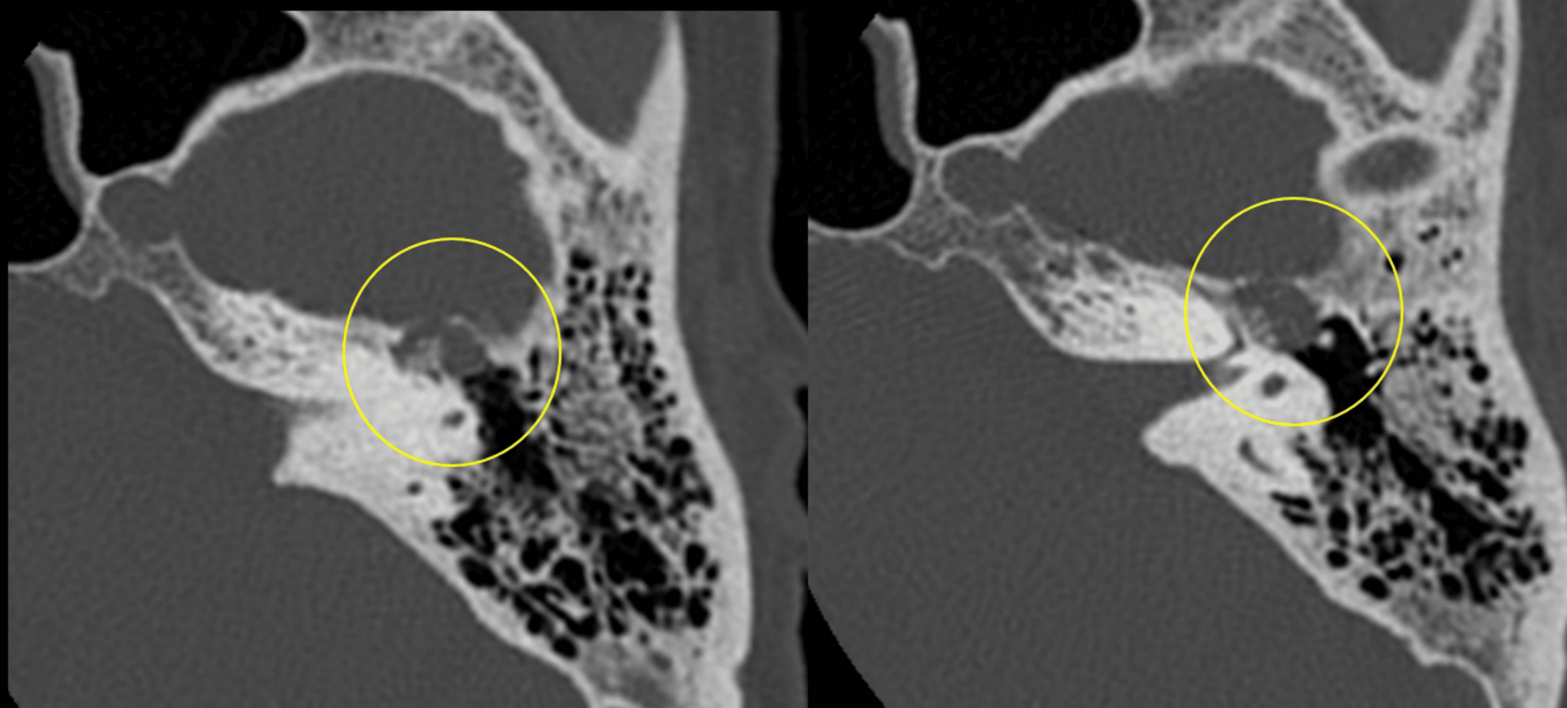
Axial thin-section CT image of the left temporal bone shows an expansile lytic lesion centered at the geniculate ganglion, containing fine punctate calcifications and delicate internal bony spicules, producing a characteristic “honeycomb” appearance (yellow circle), highly suggestive of a facial nerve hemangioma.
